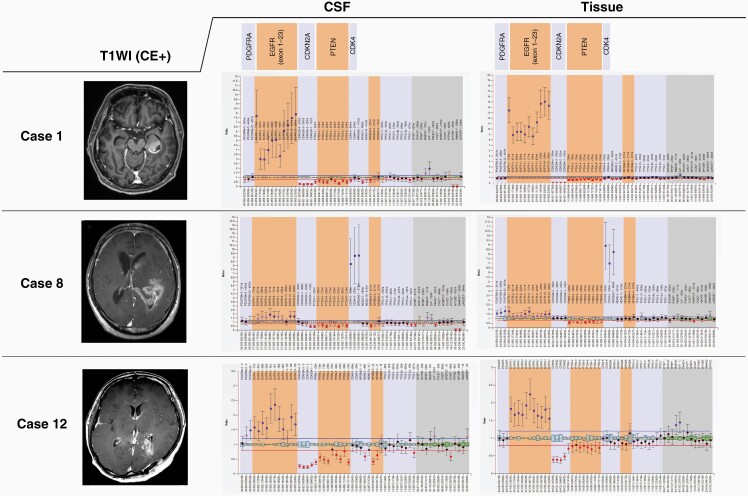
Examples of representative copy number analysis MLPA results obtained using assay P105. Ratio charts indicate relative alterations from normal PDGFRA, EGFR, CDKN2A, PTEN, and CDK4 copy numbers in that order from the left. Copy number alterations detected by MLPA using cell-free DNA from cerebrospinal fluid (left) were concordant with those detected using tumor tissue (right). Contrast-enhanced T1-weighted images of each case are also shown. CDK4: cyclin-dependent kinase 4, CDKN2A: cyclin-dependent kinase inhibitor 2A, EGFR: epidermal growth factor receptor, MLPA: multiplex ligation-dependent probe amplification, PDGFRA: platelet-derived growth factor receptor alpha, PTEN: phosphatase and tensin homolog.
